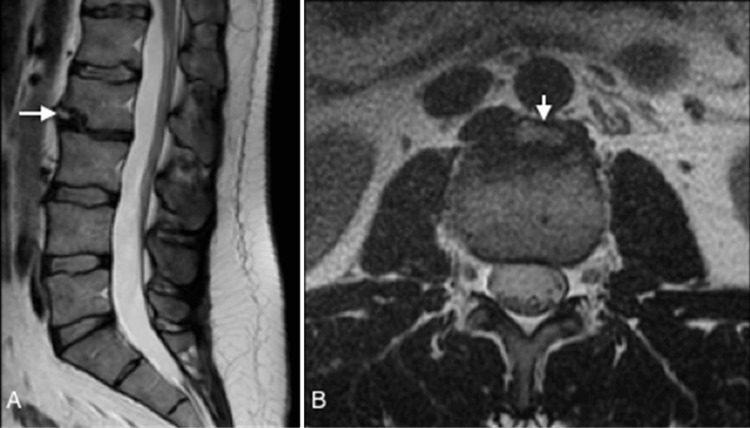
Sagittal (A) and axial T2-weighted (B) images show a detached fragment (arrow) at the level of the antero-inferior corner of the L2 vertebra, due to the intraosseous herniation of an adjacent portion of the nucleus pulposus The image credits belong to the authors.
